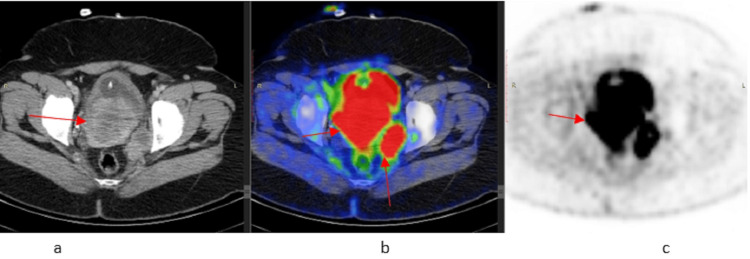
PET-CT demonstrates hypermetabolic mass involving the cervix and vagina with local infiltration of the urinary bladder (2a and 2b) along with hypermetabolic bilateral pelvic side wall lymphadenopathy (2c) PET: positron emission tomography
